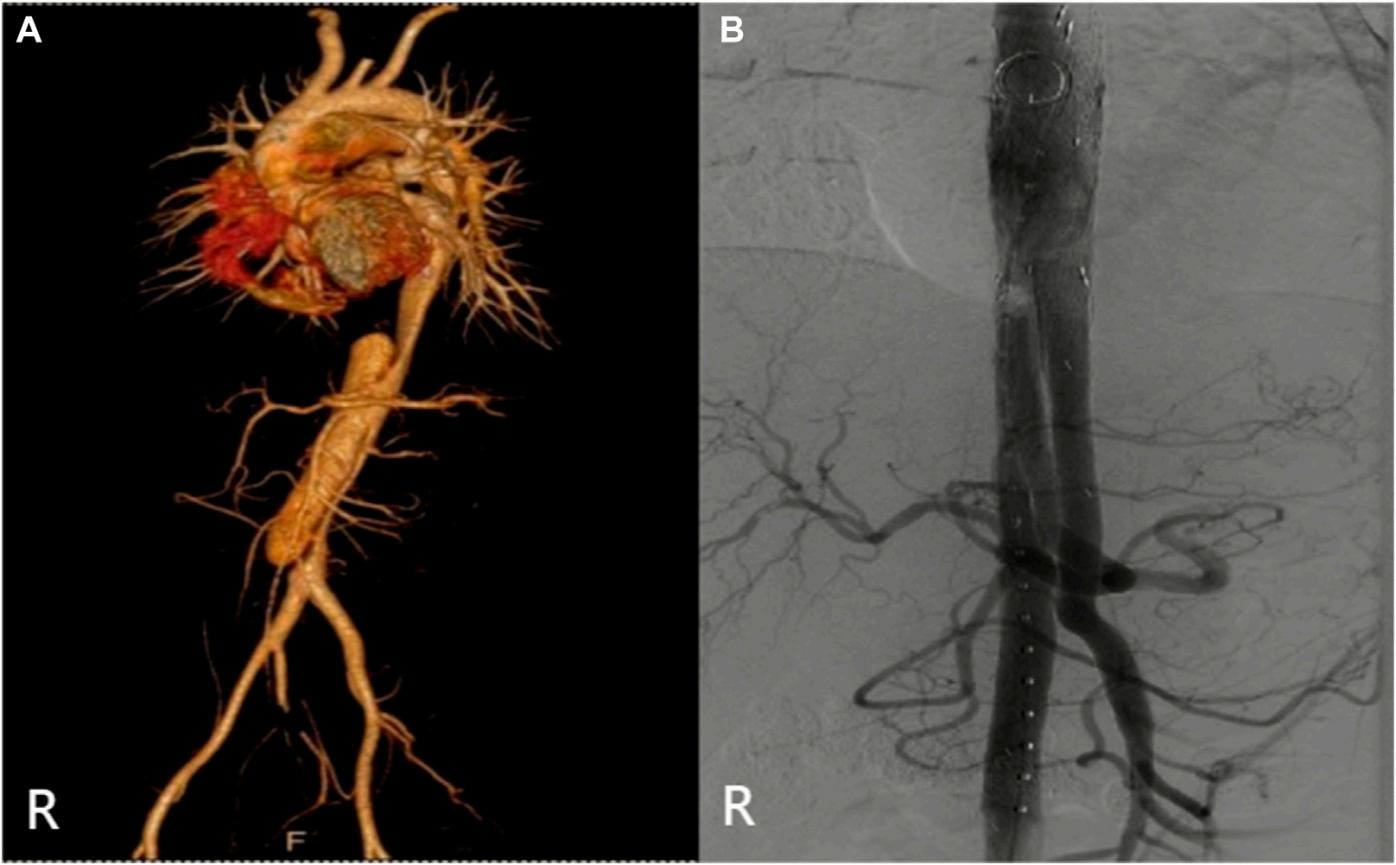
(Case 6) (A) Preoperat CTA. (B) Intraoperative imaging.
